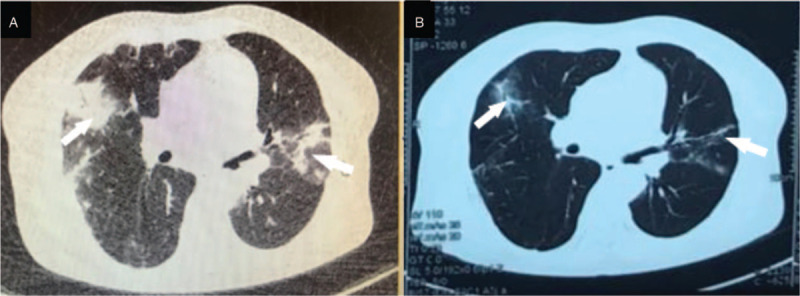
Chest CT scan showing multiple pulmonary lesions in Case 3. A, They all disappeared. B, After lenalidomide plus chidamide therapy. CT = computed tomography.
